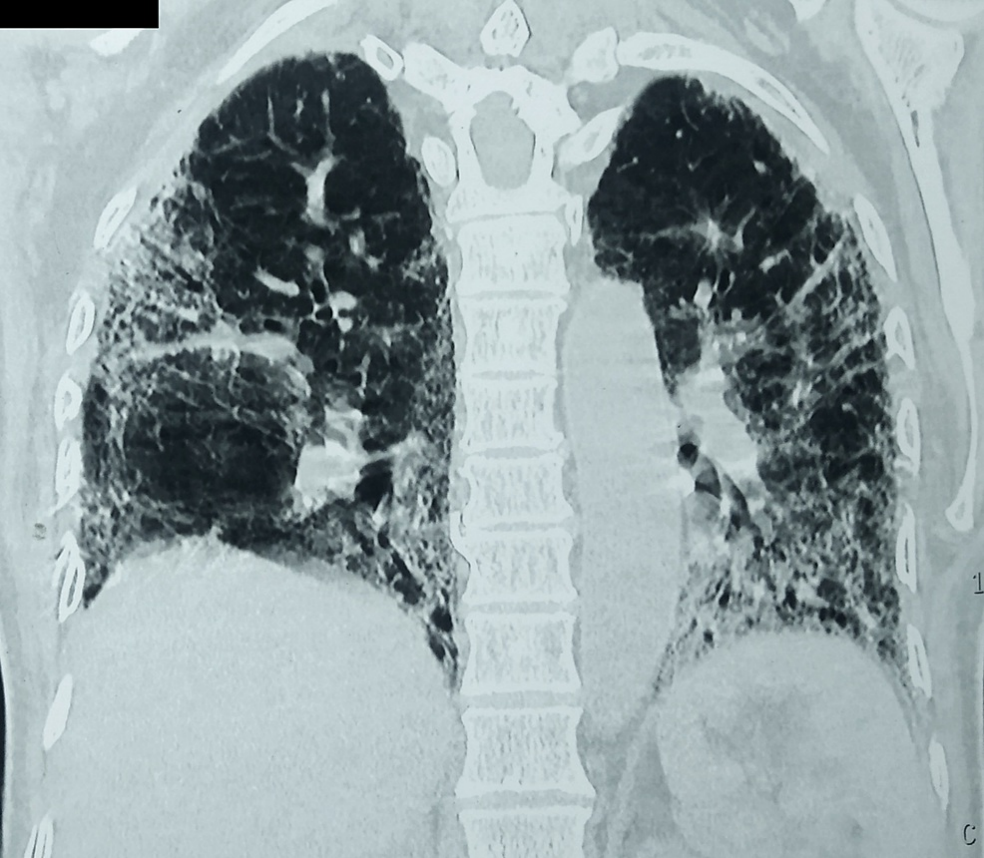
Coronal section of the high-resolution CT of the chest demonstrating bilateral ground-glass opacities CT: computed tomography
